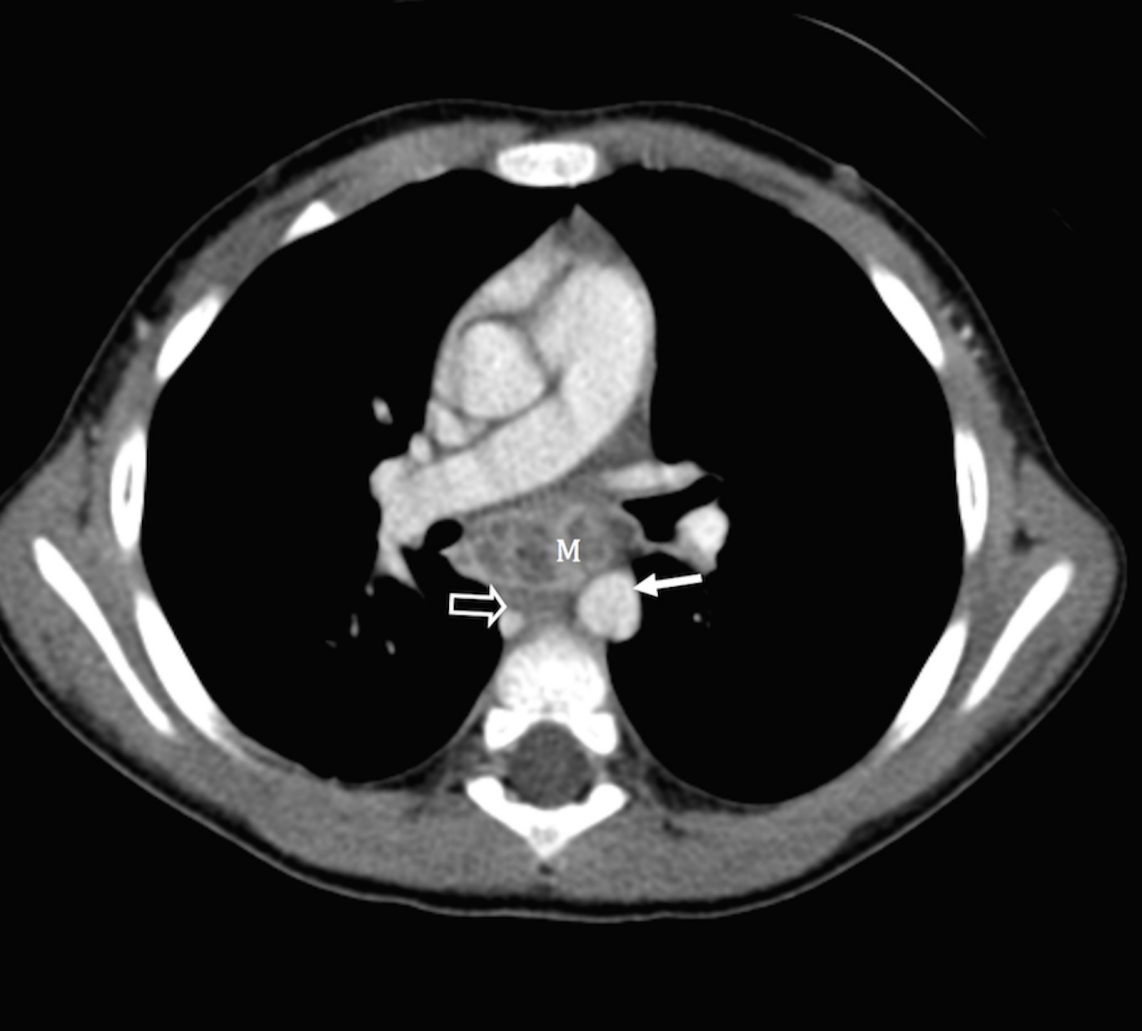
Axial post contrast CT of mediastinal mass Axial post contrast CT image in mediastinal window at subcarinal level shows a predominantly cystic peripherally enhancing subcarinal mass (M) abutting the descending thoracic aorta (arrow) and causing mass effect on the esophagus (open arrow).
